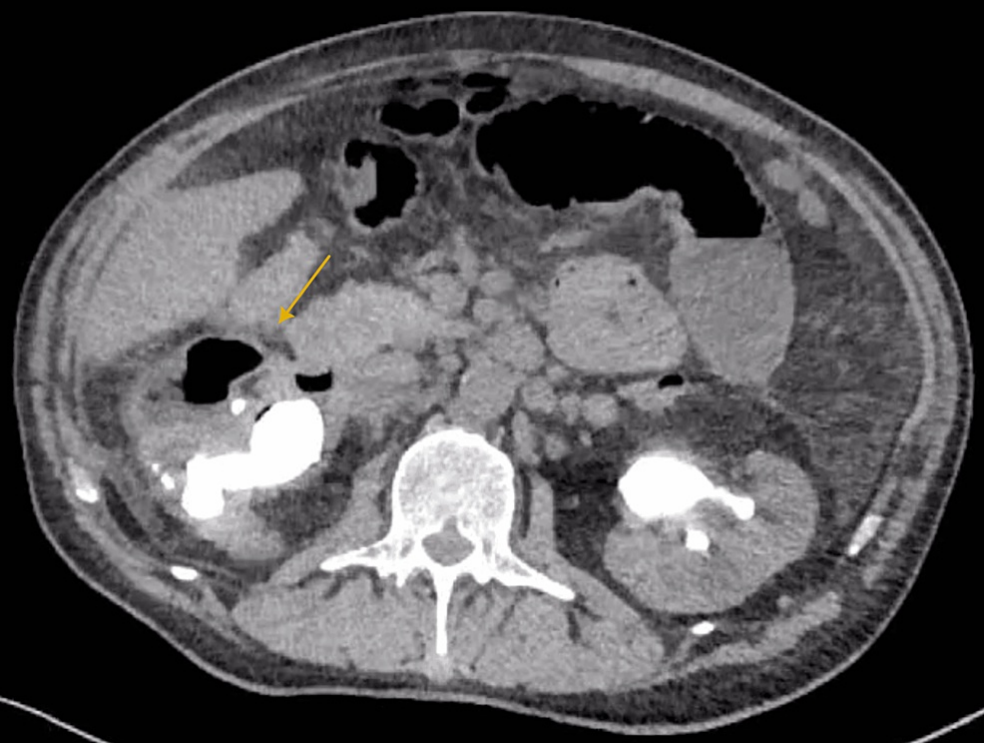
Axial plane CT KUB show the presence of bilateral staghorn stones and right emphysematous pyelitis
